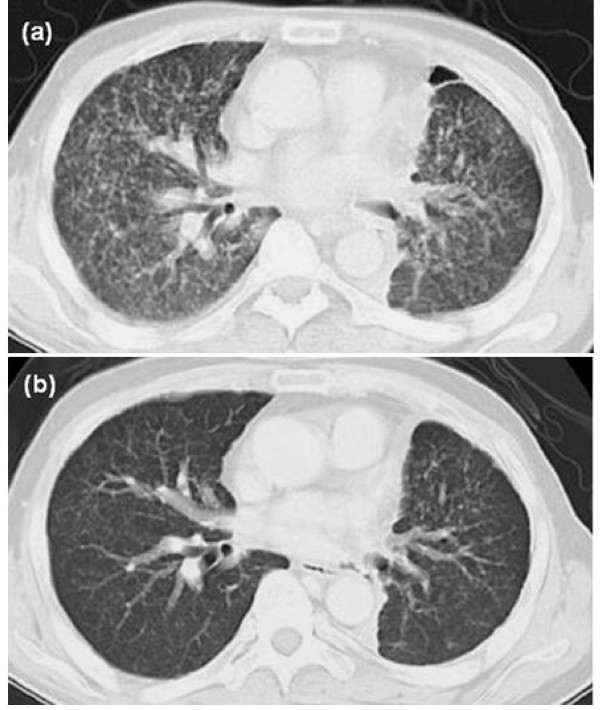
Radiological evaluation of response to re-treatment with gefitinib on CT scans. (a) before re-treatment, (b) after re-treatment.
